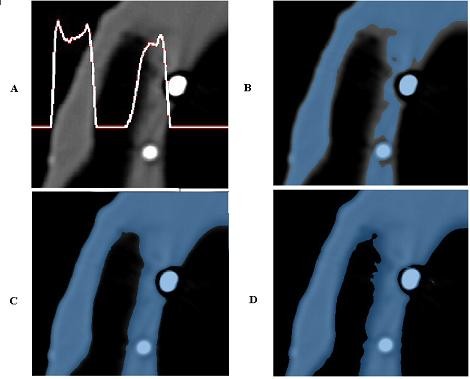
(A-D) CT data segmentation process. (A) DICOM image of the radiographic template with associated a row grey intensity level, (B-D) segmentation with three different threshold values.
